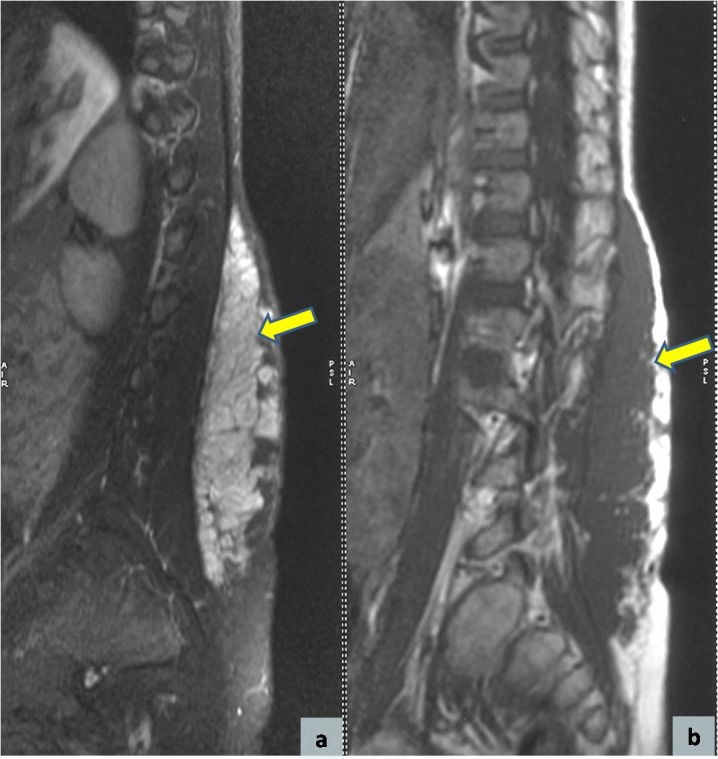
Figure 1: Magnetic resonance imaging (MRI) scans showing an elongated multinodular subcutaneous tumor (arrows), hyperintense on T2W (a) and isointense as muscle on T1W image (b).
